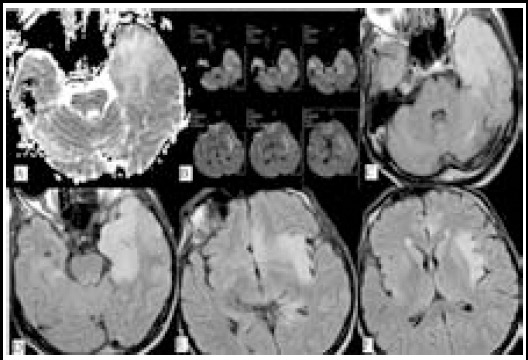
53 year-old male patient with encephalitis, on the 15^th^ day of admission: On ADC sequence (image A), DWI (image B) and axial FLAIR sequences (images C-F), hyperintensity extending from left temporal lobe up to insula and cingulate gyrus is being observed but conspicuity of signal alterations is best depicted on FLAIR images.
